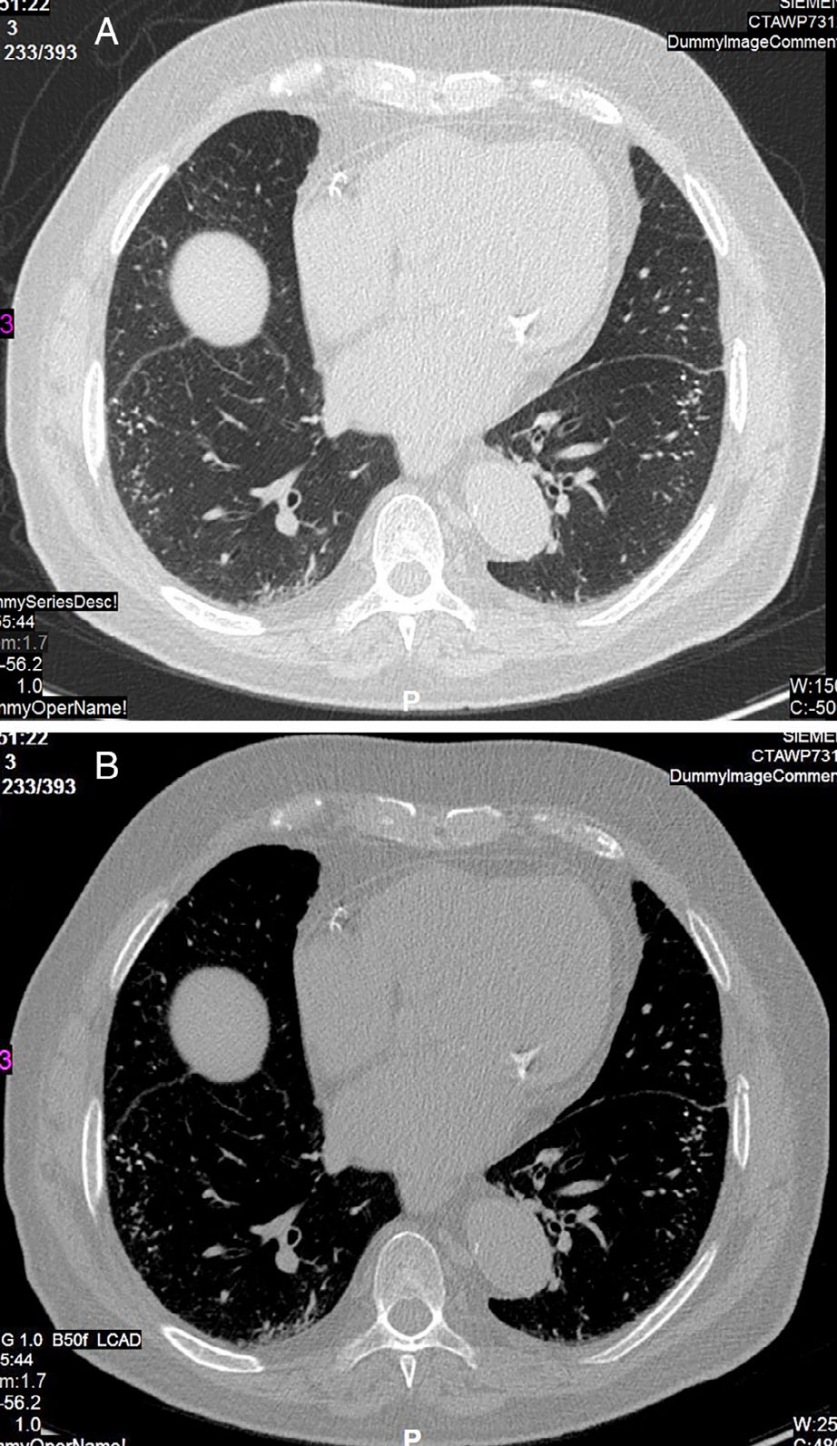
Case 2: An 83‐year‐old male with disseminated pulmonary ossification and fibrotic lung changes. (A) High‐resolution computed tomography using lung window settings demonstrated branching dendritic calcifications mainly in the lower lobes with mild reticular changes. (B) In bone windows, fine calcifications are seen
